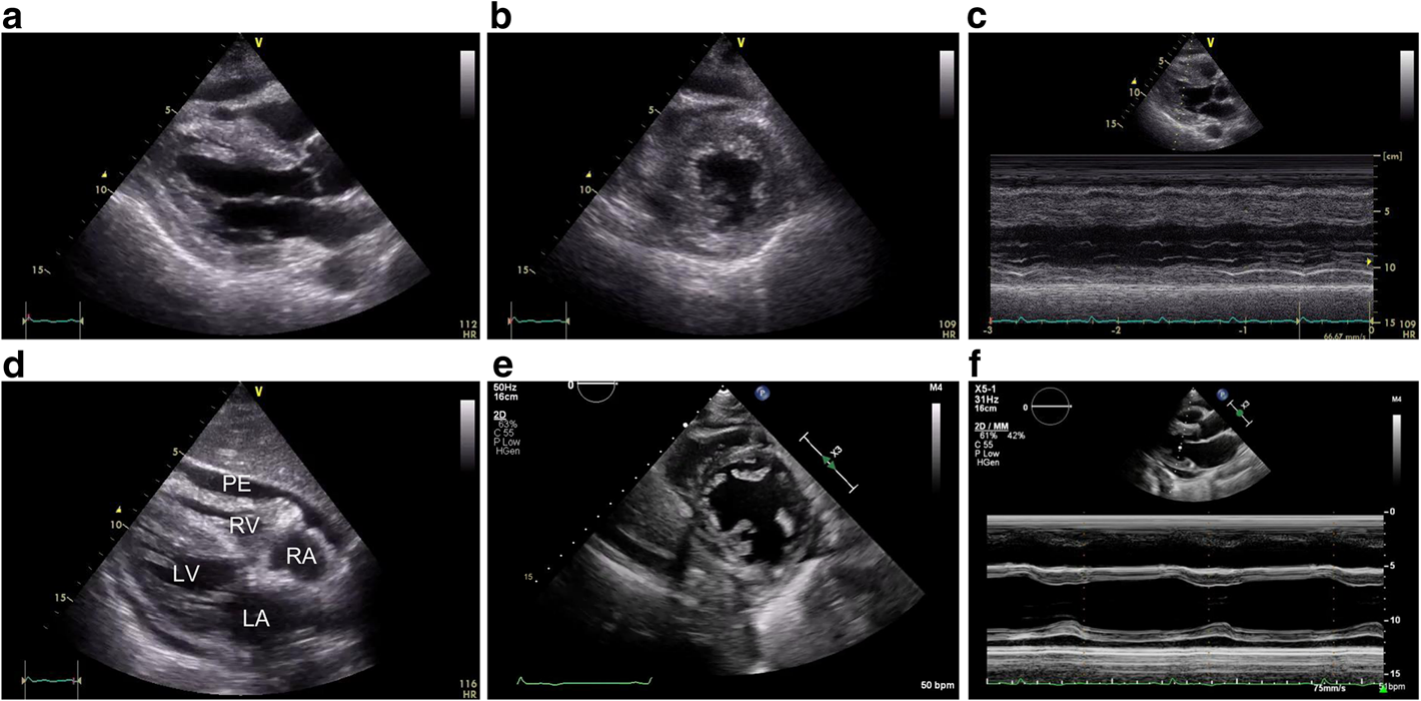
Echocardiograms on days 5 and 6, and 17. Day 5: Parasternal long- and short-axis end-diastolic views demonstrating thickening of the diffuse left ventricular wall and narrowing of the left ventricular cavity (a, b). M-mode Mesurement of left ventricle (LV) showing depressed LV contraction (c). Day 6: Subcostal four chamber view demonstrating small amount of pericardial effusion and right ventricular diastolic collapse (d). Day 17: Parasternal short-axis end-diastolic views demonstrating returned to normal wall thickness and cavity of LV (e). M-mode Mesurement of LV demonstrating normal LV contraction (f). PE: pericardial effusion; RA: right atrium; RV: right ventricle; LA: left atrium; LV: left ventricle
